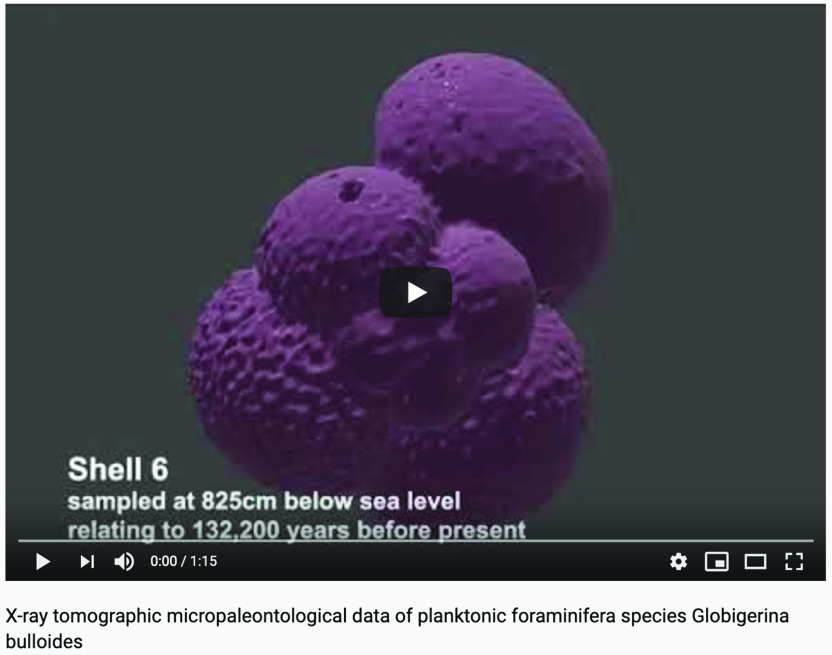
Video of example X-ray tomographic micropaleontological data *from Globigerina bulloides* sampled at three different core depths. See: https://youtu.be/gQ0VoE4YNbc
